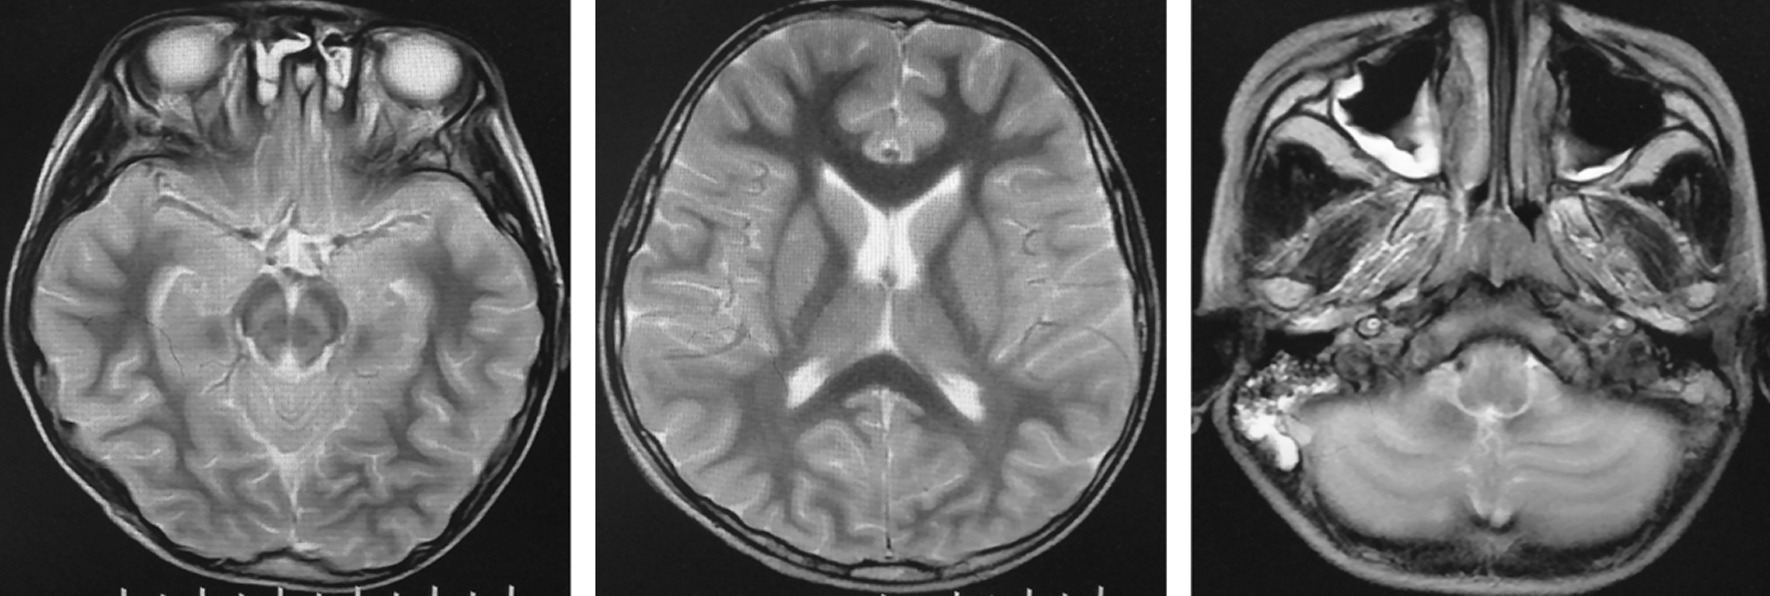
Patient head/neck MRI scans of the patient. Images exhibit diffuse swelling with abnormal signals in the bilateral cerebral hemispheres, basal ganglia, thalamus, brainstem, cerebellar hemispheres and also highlights extensive cerebral edema. The fourth ventricle, ring cistern, pontine cistern and extra-cerebral space are narrow and the lower edge of the cerebellar tonsils have become pointed and moved down slightly
